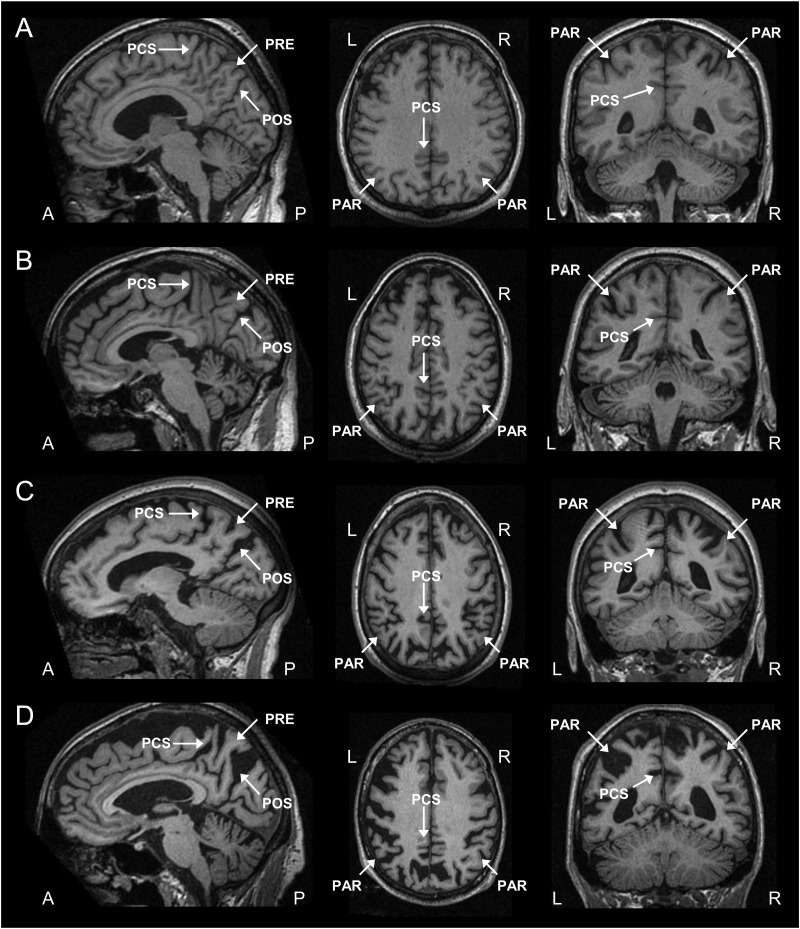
T1-weighted sagittal, axial, and coronal images as examples for each grade of the posterior atrophy (PA) scale. (A) Grade 0 = no atrophy; (B) Grade 1 = minimal atrophy; (C) Grade 2 = moderate atrophy; and (D) Grade 3 = severe atrophy. PAR, parietal lobe; PCS, posterior cingulate sulcus; POS, parieto-occipital sulcus; PRE, precuneus.
